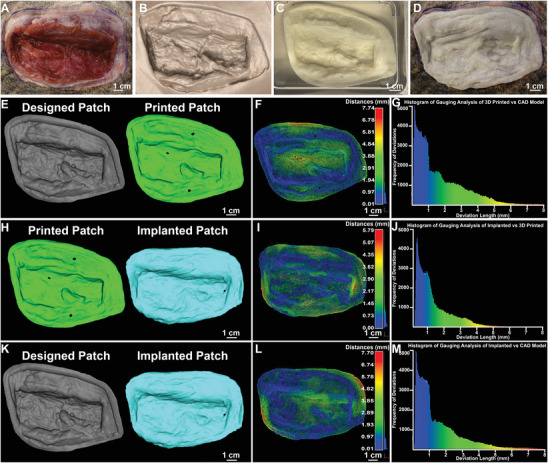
Dimensional analysis comparing the designed dECM patch model, to the FRESH printed dECM patch pre‐ and post‐implantation. A) Photograph of VML wound. B) CT scan of VML wound rendered in 3D. C) Photograph of dECM patch submerged in 50 mM HEPES solution. D) Photograph of implanted dECM patch on the wound bed. E) Original CAD model used for printing (gray) compared to the CAD model created from the CT scan of the printed patch (green). F) The color map results from gauging analysis of the printed patch compared to the original CAD model. The dark blue color indicates <0.01 mm difference, demonstrating an almost perfect fit. G) The histogram results from the gauging analysis of the printed patch compared to the original CAD model. H) The geometry of the 3D printed patch (green) was compared to the geometry of the patch post‐implantation (blue). I) Color map results from gauging analysis of the implanted patch compared to the as printed patch. J) Histogram results from gauging analysis of the implanted patch compared to the asprinted patch. K) The geometry of the original cad model (gray) was compared to the geometry of the patch post‐implantation (blue). L) Color map results from gauging analysis of the implanted patch compared to the original CAD model. M) Histogram results from gauging analysis of the implanted patch compared to the CAD mode used for printing.
